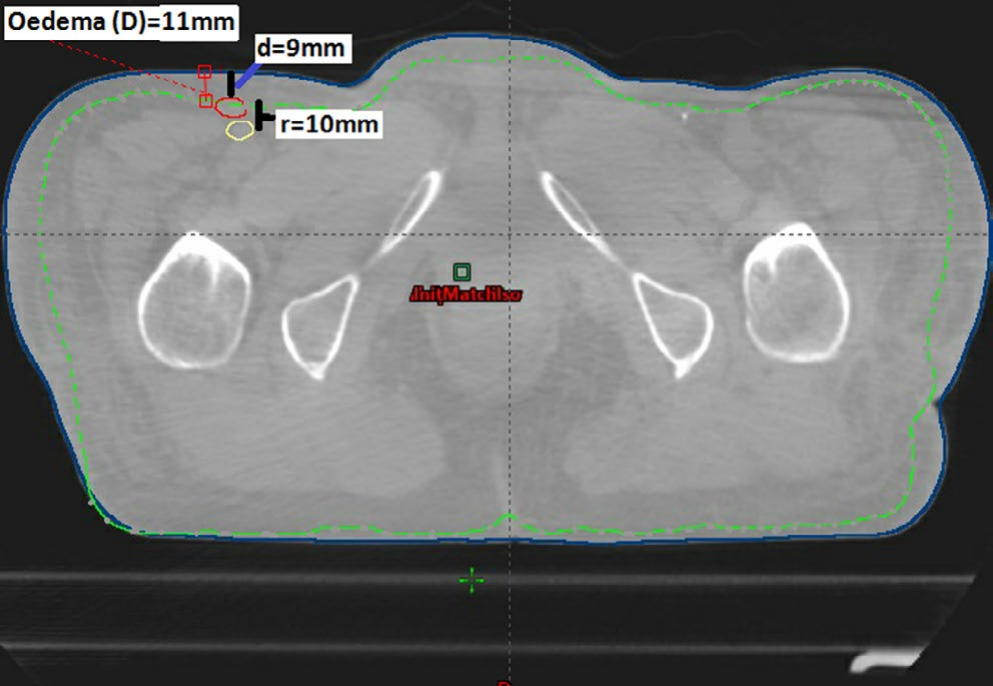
Transverse section of CBCT of fraction number 28 for the presented patient. The outer surface contour is the CBCT contour. The interrupted contour is the planning CT (pCT) body contour. The developed edema is 11 mm on the right inguinal side (difference between pCT and CBCT contours). Red and yellow contours are GTV‐N on CBCT and pCT, respectively. The geographical shift of this LN from pCT to CBCT (center of mass) (“r”) was 10 mm. The depth of this LN from the skin was 9 mm on this CBCT
